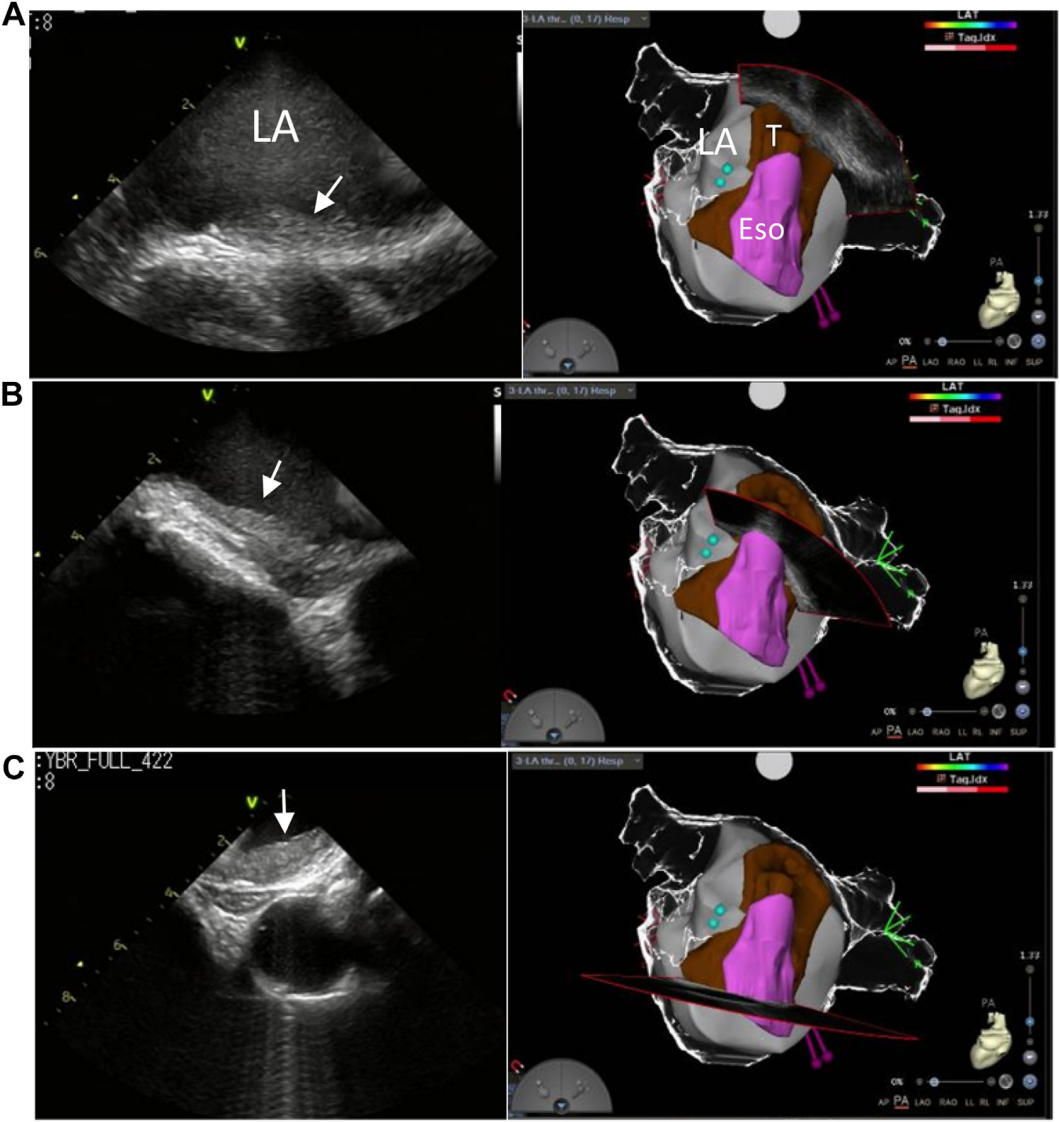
Intracardiac echocardiography from the left atrial (LA) cavity demonstrated that the hyperechoic and flat mass lesion adhered to the posterior wall (*arrows*). Transverse views at high (A), middle (B), and low levels (C) of the LA posterior wall are shown. LA posterior views of CARTO (Biosense Webster, Diamond Bar, CA) image are provided in the *right*. *Brown color* indicates thrombus and *pink color* indicates esophagus.
